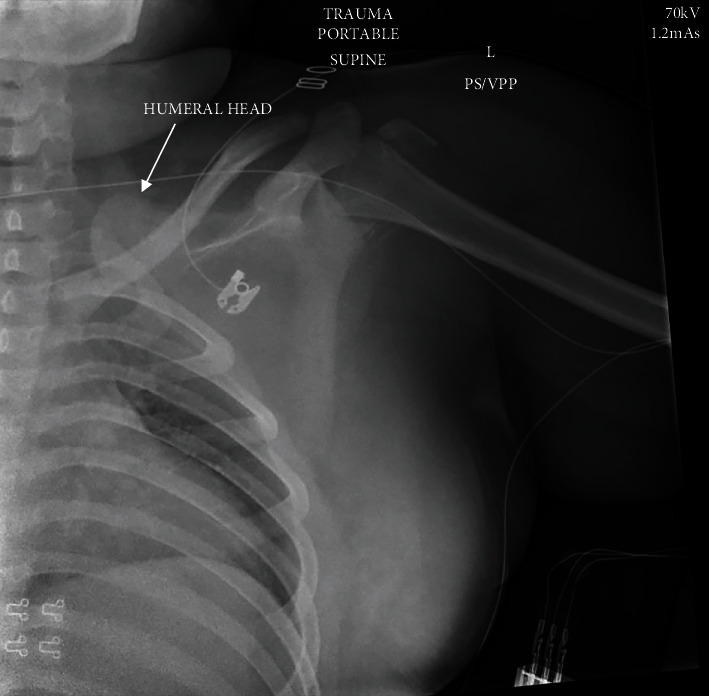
Preoperative anteroposterior X-ray of the left shoulder showing proximal humerus fracture with the humeral head sheared off and displaced medially in the region of the first rib and medial clavicle. Comminuted fragments are noted around the proximal humeral metaphysis.
